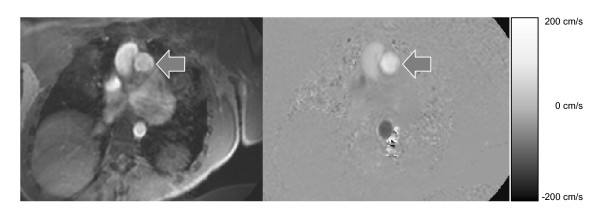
The method for measuring flow in a vessel using cardiovascular magnetic resonance imaging. The left panel shows the modulus reconstructed phase contrast image in a cross section of the pulmonary artery (arrow). The right panel shows the corresponding phase reconstructed image. The scale to the right shows the velocities in the through-plane direction. Figure 3A shows the corresponding flow in the pulmonary artery.
